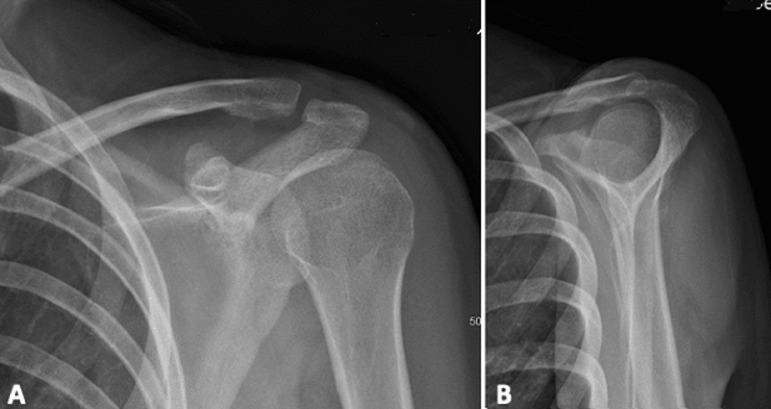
A) post-operative X-rays on day 45, AP; B) and lateral view
